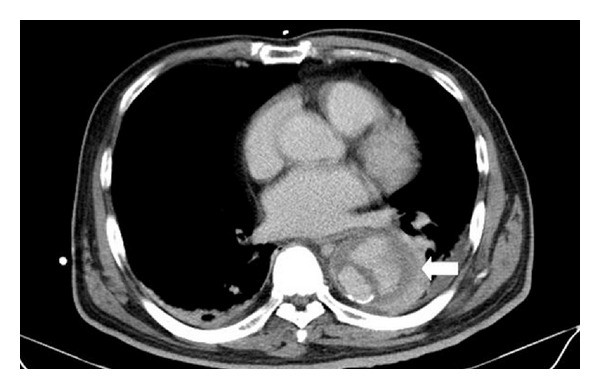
Computed tomography of chest showed progressive dilated aorta and enlarged intramural hematoma (arrow).
